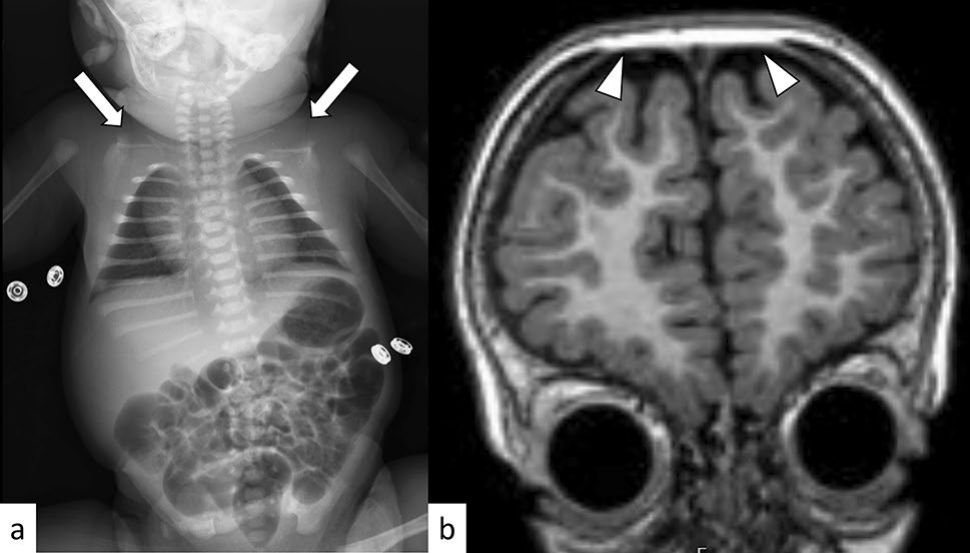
A female neonate a and a 2-year-3-month-old male b with CCD. Plain radiograph shows an absence of the bilateral clavicles (a, arrows). T1-weighted coronal image shows widened open anterior fontanelle (b, arrowheads)
